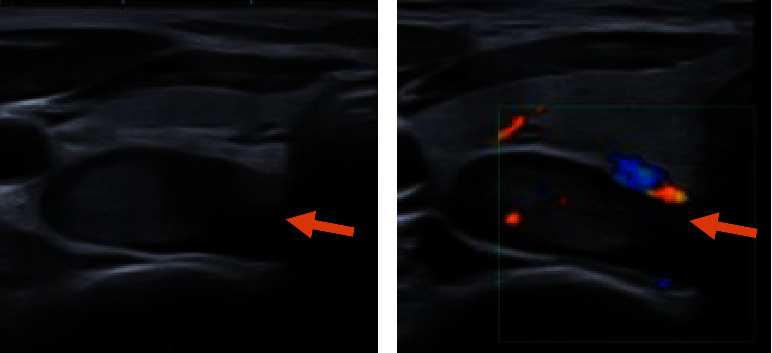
The ultrasound from the patient with a giant parathyroid adenoma. Transverse grayscale images (a) show a giant parathyroid adenoma with a thick capsule and internal echogenic components (arrow). Transverse color doppler images (b) show minimal detectable internal flow (arrow).
